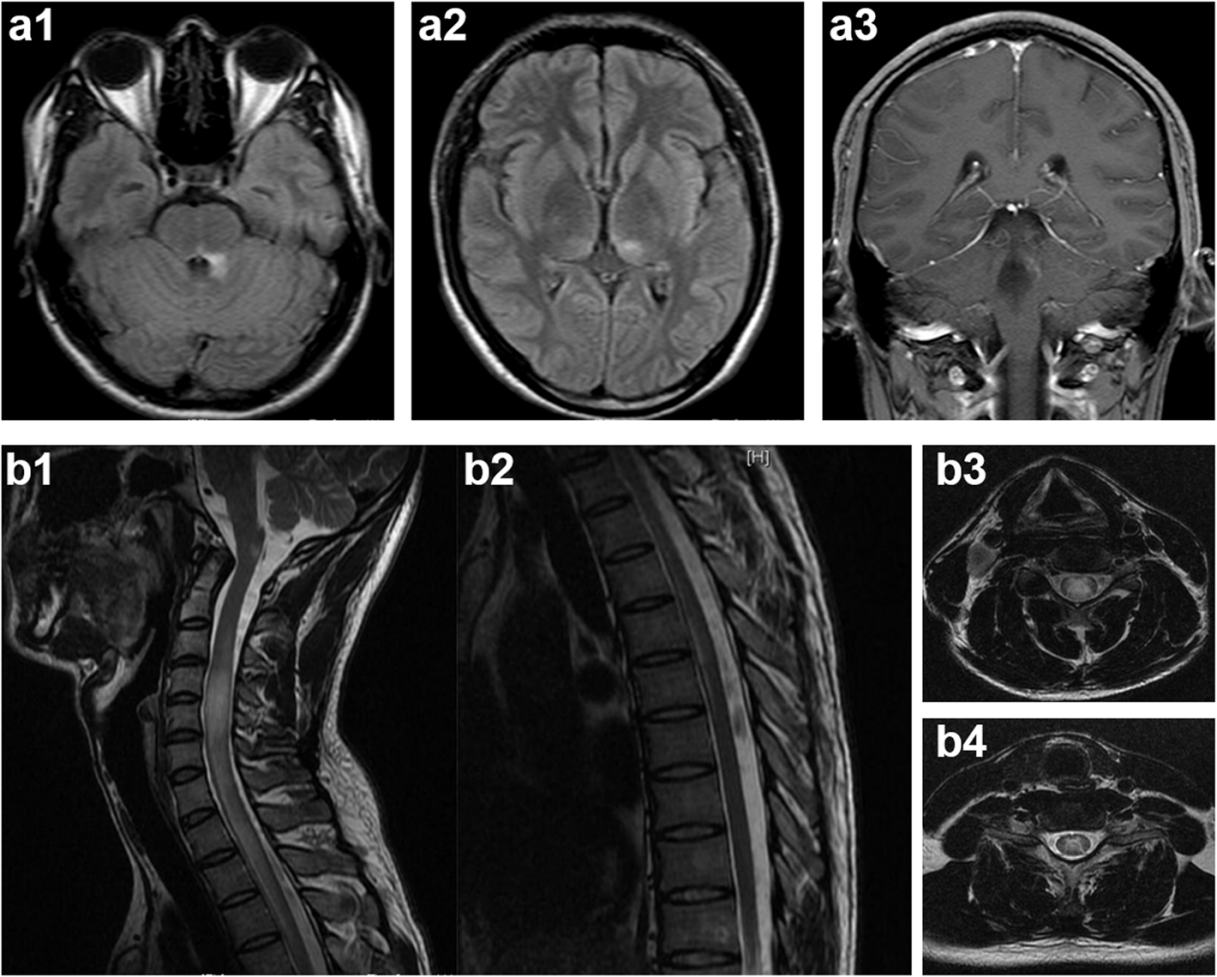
MRI findings of the patient during the second episode. a1, a2: Flake-like hyperintense signal lesions located in the dorsal pons and left medial thalamus in T2-FLAIR. a3: Enhancement in the dorsal pons. b1, b2: Lesions in the area postrema and C3–T6 levels of the spinal cord. b3, b4: Lesions were mainly located in the central gray matter of the spinal cord
